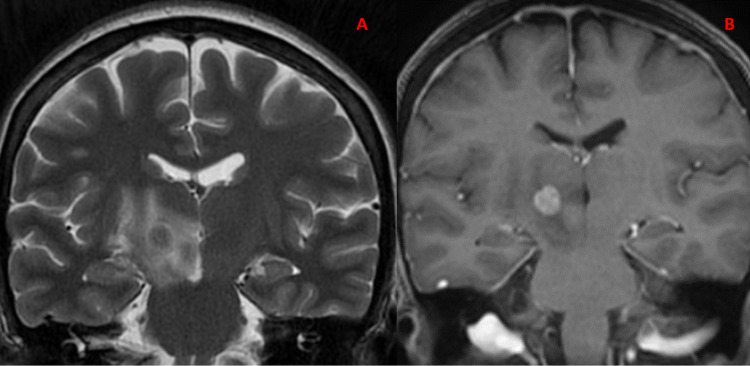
The brain magnetic resonance imaging with contrast shows a lesion of the right thalamic region surrounded by an edema. There is a annular enhancement after injection of the contrast product. (A) T2 coronal section of the brain MRI and (B) enhanced coronal section of the brain MRI.
